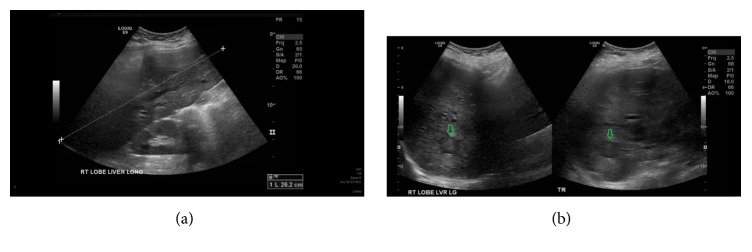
Liver ultrasound. (a) demonstrates the size of the liver measured to be about 26.2 cm. (b) demonstrates the hypoechoic mass in the right hepatic lobe (green arrows). The mass measured 2.8 cm.
